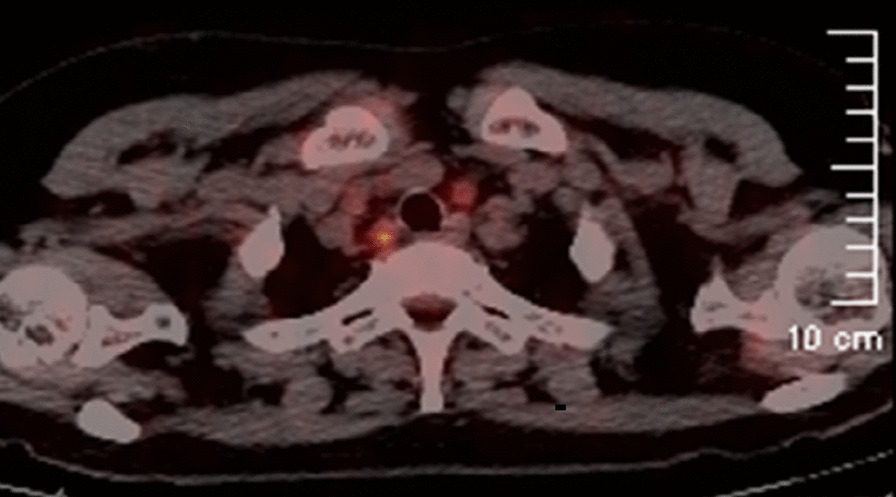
PET scan images showed a hypermetabolic lesion in the bilateral tracheoesophageal groove with an SUVmax of 13.67, which indicated the metastasis of lymph nodes
